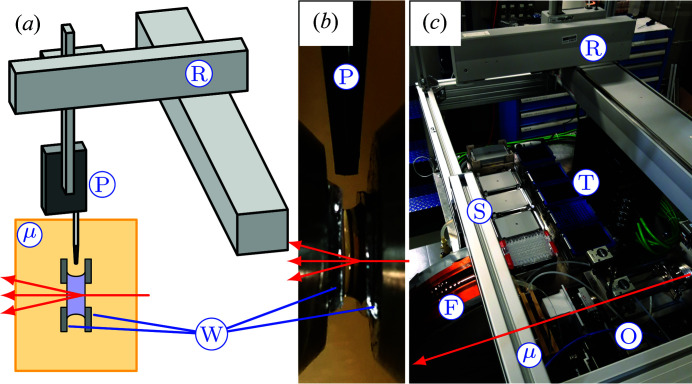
Working principle and overview of the µDrop Sample Changer. (*a*) Scheme of the instrument: the robotic arm (R) is equipped with a pipetting mechanism (P) that loads samples into the µDrop cell (µ). The drop is placed between two silicon plates (W) with X-ray-transparent windows, where it is held by surface tension. The X-ray beam (shown in red) passes through the windows, probing the sample. (*b*) Picture of a drop placed between the silicon nitride windows by pipetting from above (P). (*c*) Overview picture of the µDrop Sample Changer setup at the beamline. The X-ray path is shown by the red arrow. The robotic arm (R) takes samples from the sample trays (S) and moves them to the µDrop cell (µ). After the measurement the cell is automatically cleaned by dedicated hardware and the robot then places the next sample using a new tip taken from the tip trays (T).
